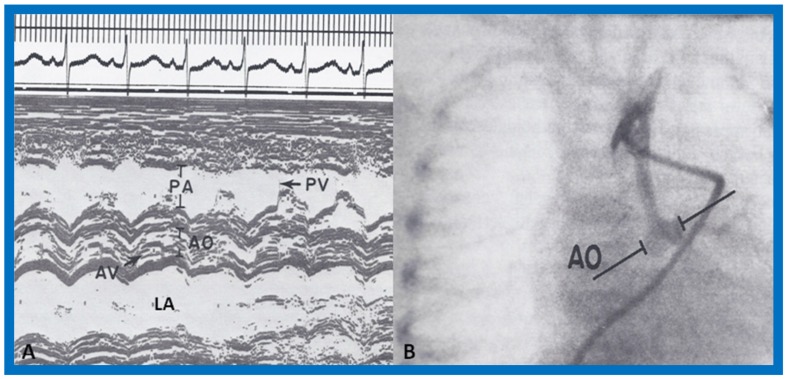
(A) Selected M-mode recordings from the parasternal short axis view of the left atrium (LA), aorta (Ao), and pulmonary artery (PA), demonstrating near normal sized aorta in a baby with hypoplastic left heart syndrome. (B) Selected cine frame from aortic arch angiogram in postero-anterior projection of the same infant shown in A, demonstrating hypoplastic ascending aorta and seemingly normal aortic sinuses, explaining the finding of near normal sized aorta in A. AV, aortic valve; PV, pulmonary valve. Modified from Covitz W, Rao P.S., et al. [4].
